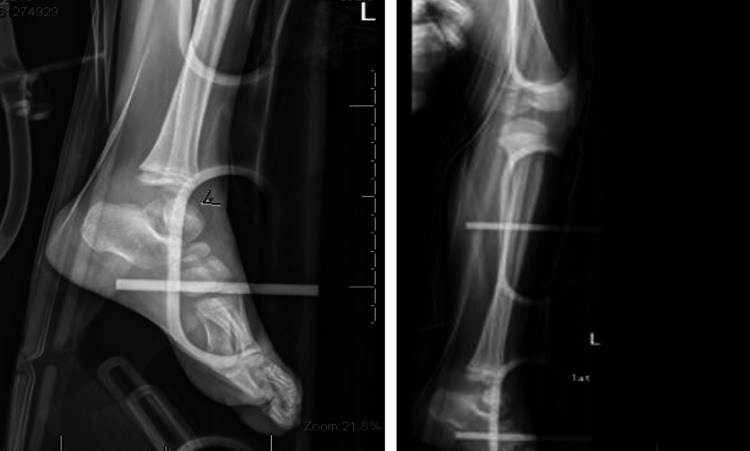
X-ray of left ankle and leg
